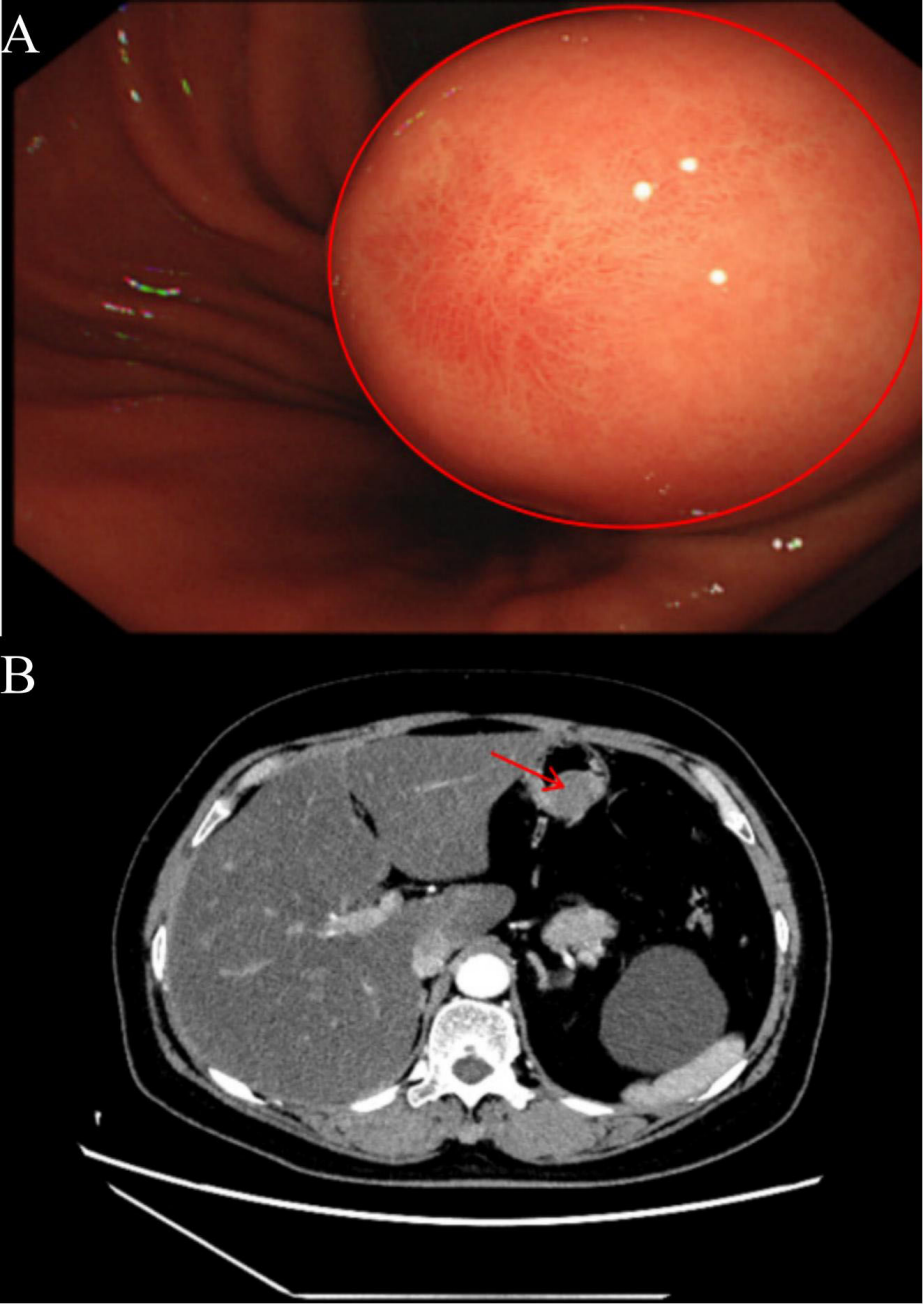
(A) The red circle indicates the gastroscopic image of the patient, revealing a significant submucosal mass in the posterior wall of the lower gastric body, characterized by limited mobility and a firm texture. (B) The red arrow indicates the tumor’s location in the upper abdomen as demonstrated by the enhanced CT scan, highlighting the arterial phase enhancement, with the tumor protruding into the gastric lumen.
